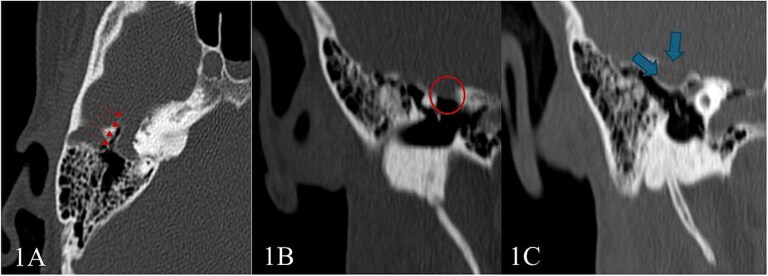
High-resolution CT scan of the temporal bone shows: (A) Tubular lucency noted lateral to superior semicircular canal with associated dehiscence. (B and C) Scalloping and protrusion of right tegmen tympani into epitympanum with a marked narrowing of epitympanum recess. Additionally, there is a lateralization of ossicular chain and apparent subluxation of incudomalleolar joint.
